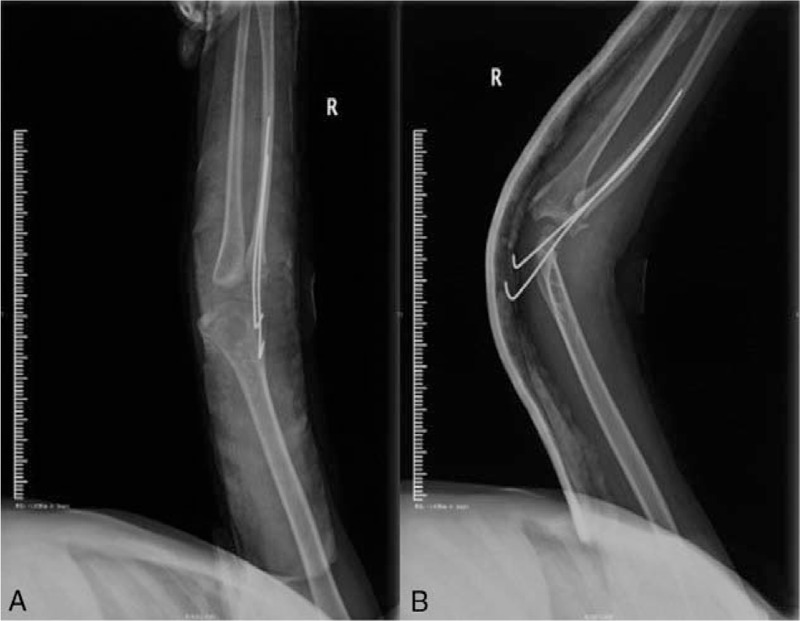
(A) Immediate postoperative X-ray of the anteroposterior elbow. (B) Immediate postoperative X-ray of the lateral elbow.
